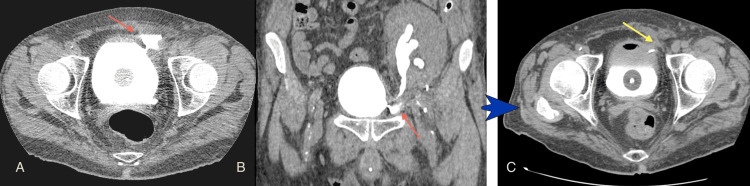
Urine leak of the anastomosis close to the bladder (A) Axial image and (B) Coronal multidetector computed tomography (MDCT) projection show contrast medium outside the urinary system at the entry of the ureter in the bladder (red arrows); (C) After conservative treatment, the follow-up retrograde MDCT urography shows no leakage around the ureteric stent (yellow arrow). Image source: Department of Radiology, Ippokrateio General Hospital of Thessaloniki. Permission obtained for reproduction.
